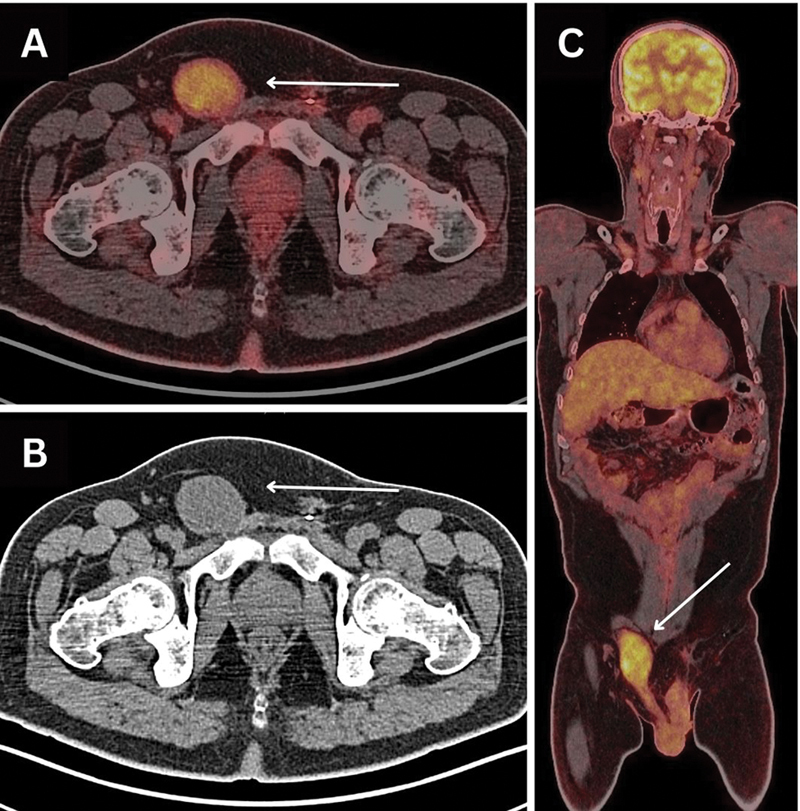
Whole-body positron emission tomography/computed tomography (PET/CT) scan by fluorodeoxyglucose (FDG) (
